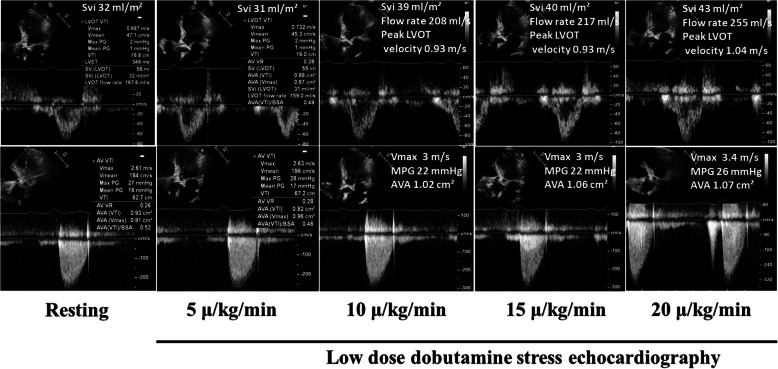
Changes in aortic flow, peak LVOT velocity, MPG, Vmax and AVA from rest to peak dobutamine stress echocardiography. AVA, aortic valve area; LVOT, left ventricular outflow tract; MPG, mean aortic pressure gradient; SVi, stroke volume index; Vmax, peak aortic jet velocity
